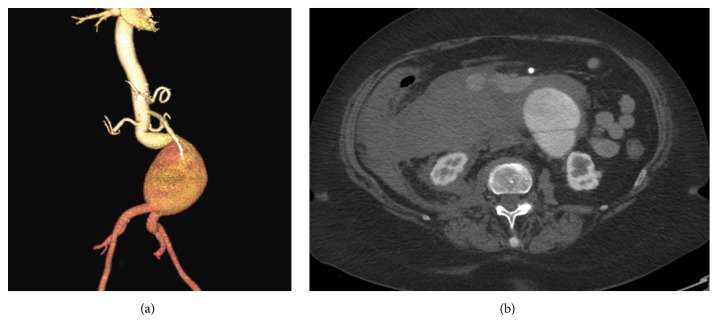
Computed tomography angiogram of ruptured AAA at initial presentation to the emergency department. (a) Three-dimensional reconstruction of the aneurysm sac. (b) Corresponding cross-sectional demonstrating rupture of AAA with active extravasation of contrast into the retroperitoneum.
